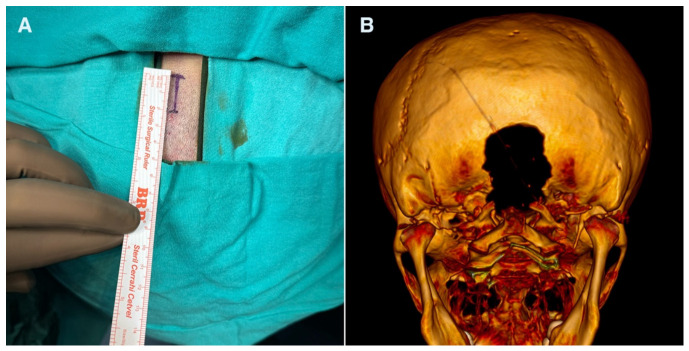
(A) Skin incision measuring approximately 2 cm. (B) Postoperative three-dimensional CT reconstruction demonstrating the extent of bony decompression.
